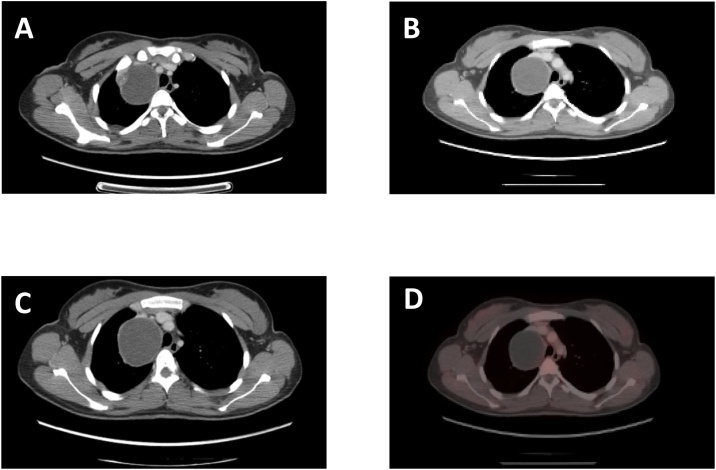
Chest CT in axial views of the heterogeneous right side anterior lateral mass (A, B,C); Chest PET of the mass (D).
